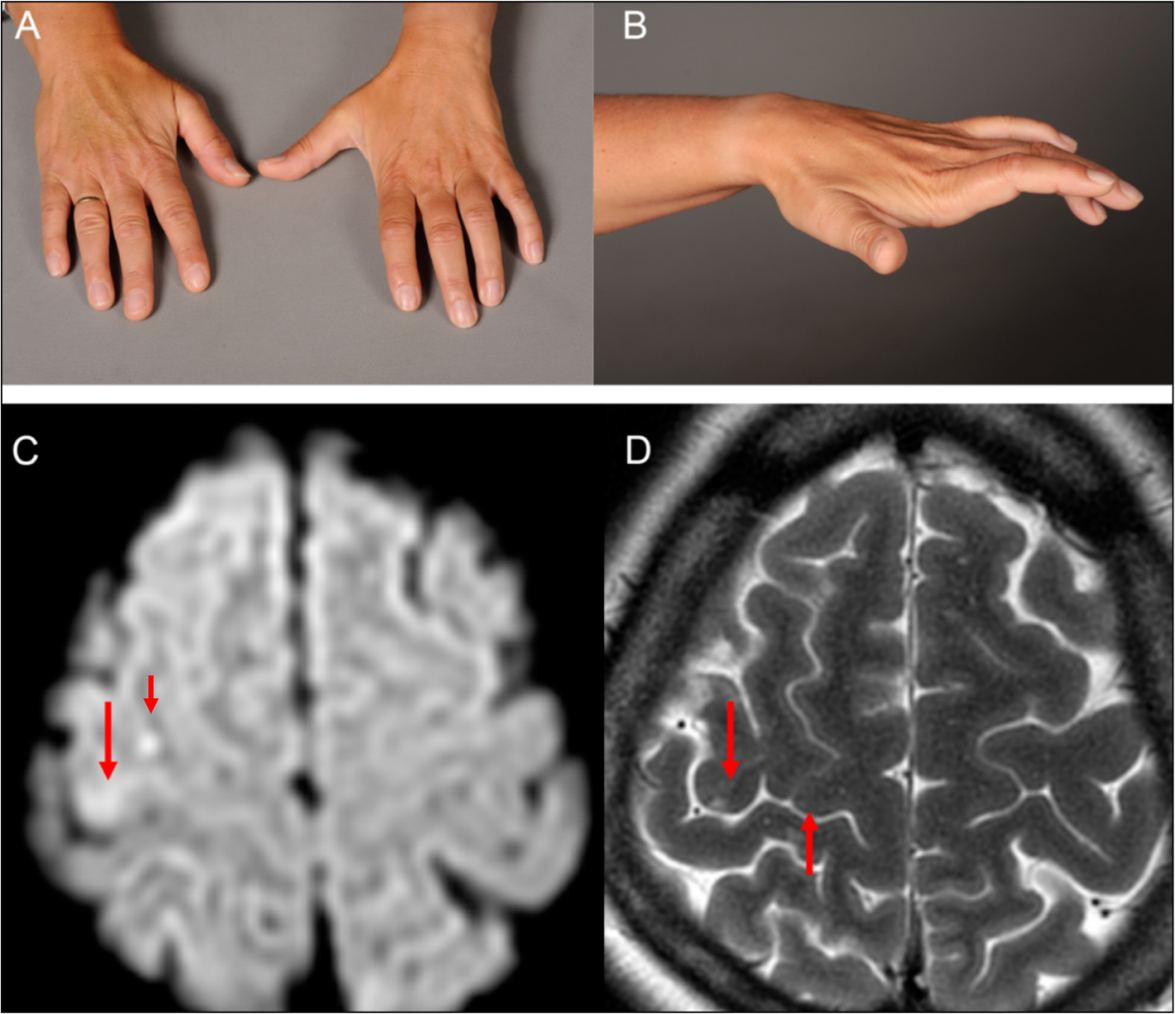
Clinical photographs of the left hand and MRI of the brain. a, b. Clinical photographs showing claw hand deformity due to infarct in the hand knob area. c. Axial diffusion- and, d, T2-weighted MRI showing hyperintense signals (upper arrows on c) in the right precentral gyrus near the central sulcus (lower arrow on d), indicating an infarct in the hand knob area (upper arrow on d)
